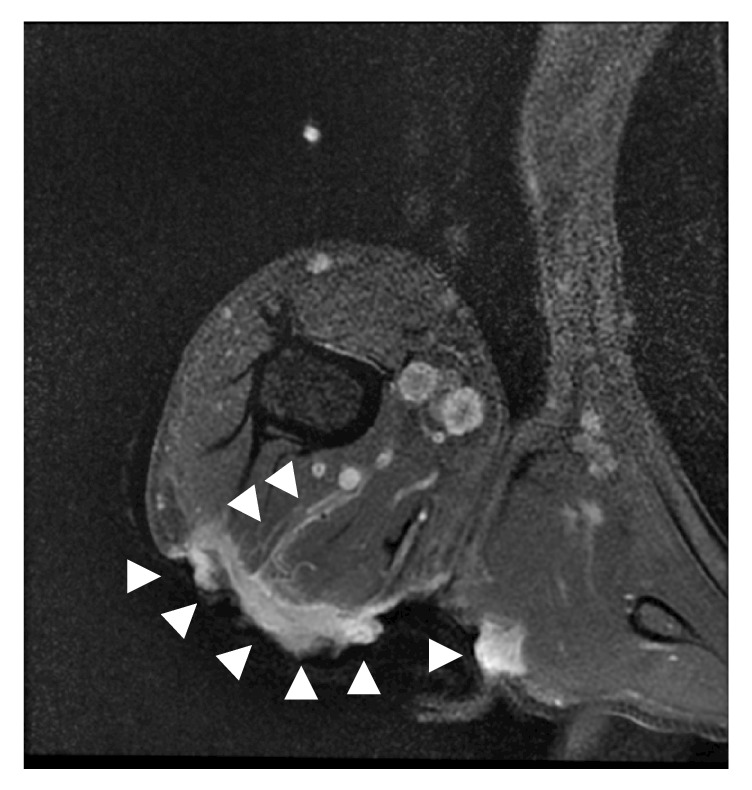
Axial view of the dermatofibroma (arrowhead) on magnetic resonance imaging; invasion to the deltoid and triceps brachii muscles is seen.
